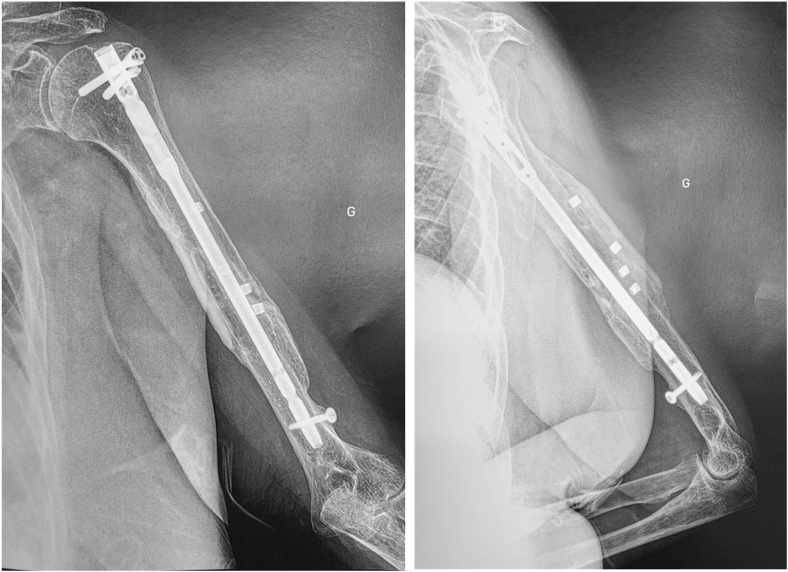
Anteroposterior and lateral radiographs at 12 months post-operatively demonstrating complete fracture consolidation and bony incorporation of circumferential cortical strut allografts following treatment of humeral shaft nonunion with exchange intramedullary nailing.
